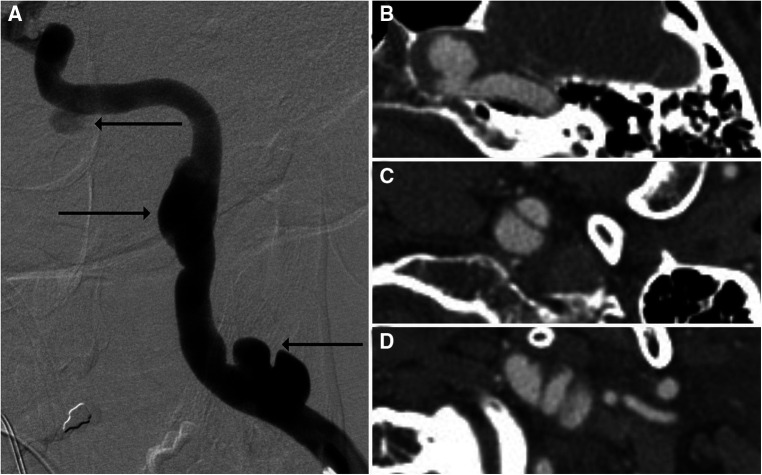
Imaging of a 52-year-old man who presented with headache. DSA (A) and CTA (B–D) showed multiple pseudoaneurysms related to previous dissection involving the left cervical and petrous internal carotid artery segments, with arrows on DSA corresponding to CTA slices.
